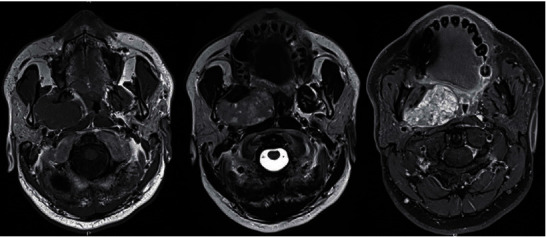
Facial MRI in axial sections weighted T1, T2, and T1 injected (from left to right): prestyloid parapharyngeal tumor independent of the parotid deep lobe with heterogeneous T2 hypersignal and enhancement after gadolinium injection.
